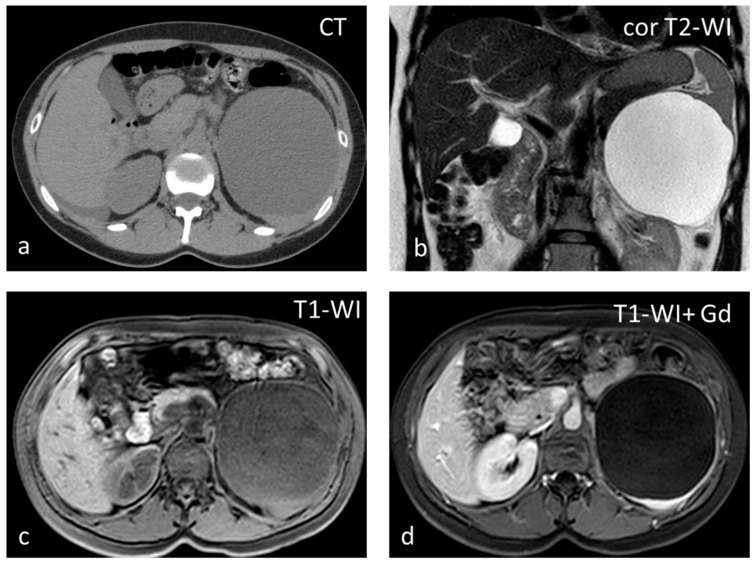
Large splenic cyst appearing homogeneously hypodense on non-contrast CT (a). The cyst content is homogeneously hyperintense on T2-WI (b) and hypointense on T1-WI (c). The cyst wall does not show significant enhancement (d).
